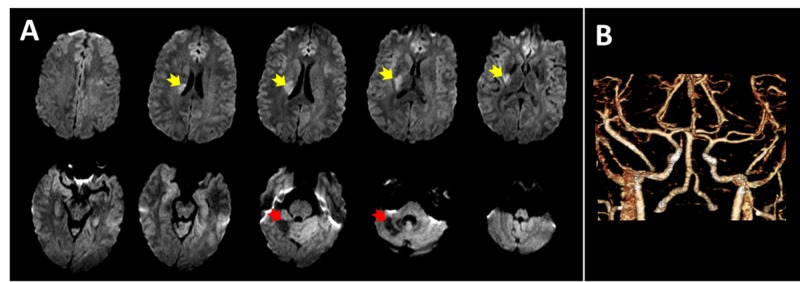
Imaging of the brain and vasculature A) Diffusion-weighted imaging of the brain demonstrates acute ischemia in the right periventricular white matter, internal capsule, and basal ganglia (yellow arrows). The patient’s prior stroke in the right cerebellum was also seen (red arrows); B) No focal stenosis, occlusions, or other vasculopathy was identified on computed tomography angiography.
